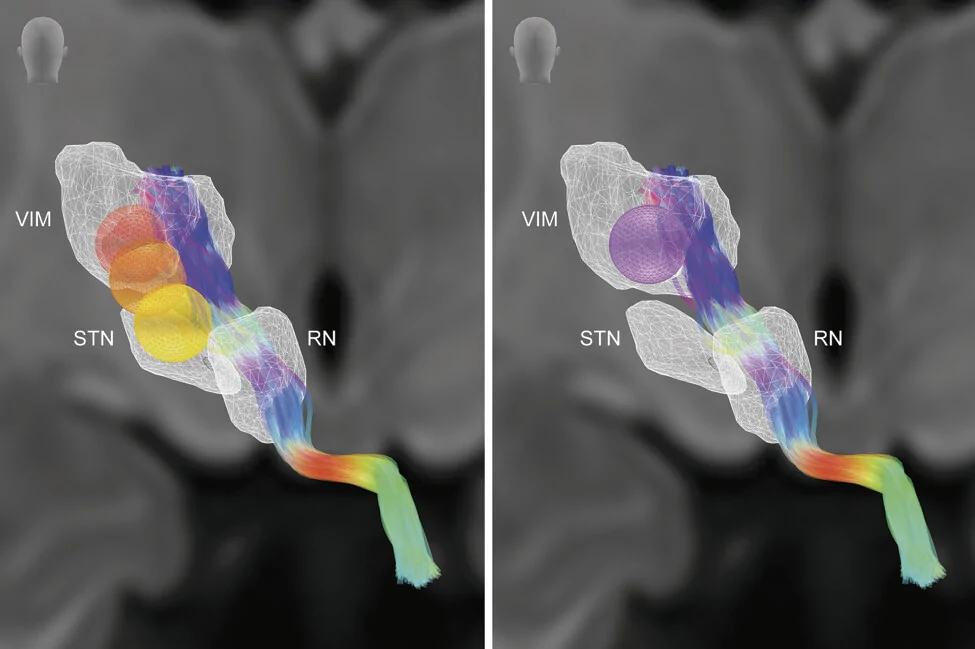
Averaged stereotactic targets for tremor therapy (deep brain stimulation [DBS] vs. MR-guided focused ultrasound [MRgFUS]). Commonly used DBS stimulation sites (left) for tremor control within the classical ventral intermediate nucleus (VIM) target (red circle). Posterior subthalamic area (PSA, yellow) and zona incerta (ZI, orange) are the two white matter targets within a stronger tremor, located close to the dentatorubrothalamic tract (DRTT, rainbow-colored streamlines). The MRgFUS (right) thalamotomy lesion centers inside the VIM (purple circle). RN, red nucleus; STN, subthalamic nucleus.
